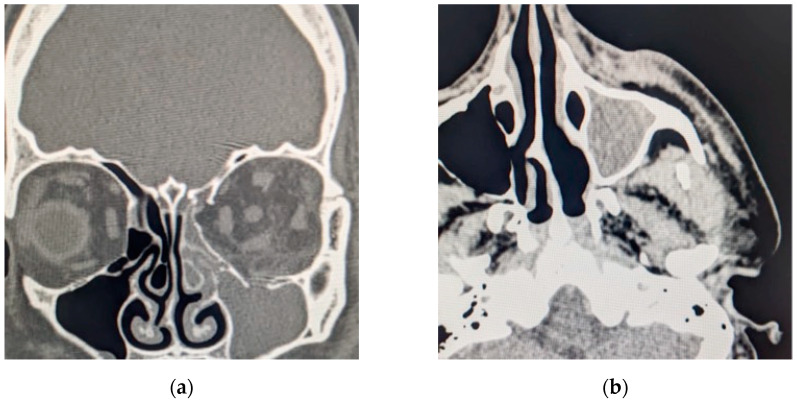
Computed tomography scan section displaying (a) coronal section with maxillary sinus opacification and orbital abscess; (b) axial section with maxillary sinus opacification.
